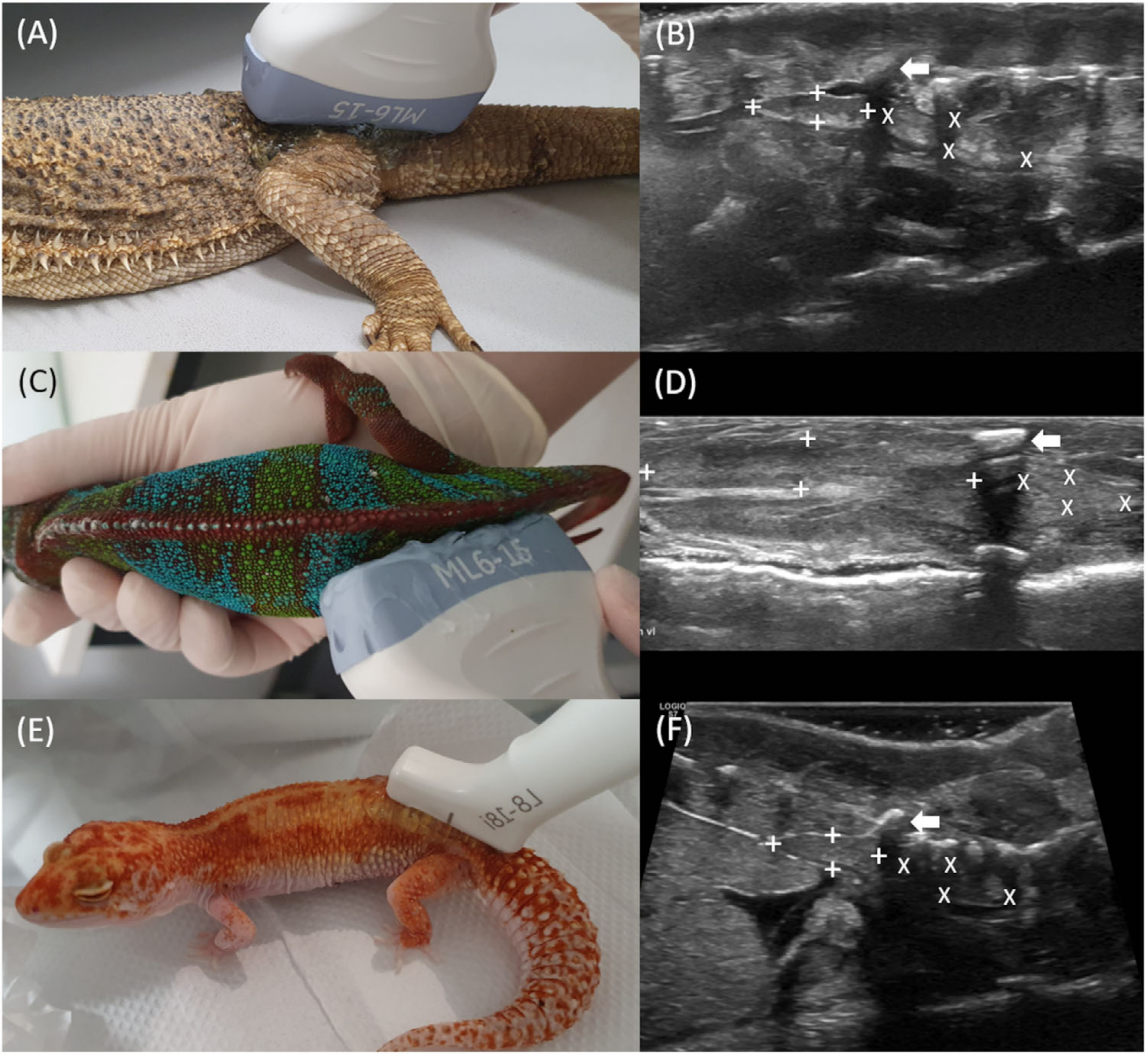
Transducer positions for the renal ultrasound and the corresponding ultrasound images in different lizard species. The cranial pole (boundaries marked by crosses) and the caudal pole (boundaries marked by Xs) are separated by the sound extinction of the hip bone (left arrow). A, Parasagittal renal sound in a bearded dragon with a 6–15 MHz linear transducer. The center is located dorsally at the level of the hip joint. B, Parasagittal ultrasound image of the left kidney of a bearded dragon. The caudal pole is not always clearly visible. C, Lateral kidney ultrasound of a panther chameleon with a 6–15 MHz linear transducer. The center is located laterally about one centimeter in front of the pelvis. D, Lateral ultrasound image of the left kidney of a panther chameleon. The caudal part is clearly smaller than the cranial part. E, Parasagittal kidney ultrasound in a leopard gecko with an 8–18 MHz linear transducer. The center is located dorsally at the level of the hip joint. F, Parasagittal ultrasound image of the left kidney of a leopard gecko. The caudal pole is partially not clearly visible.
